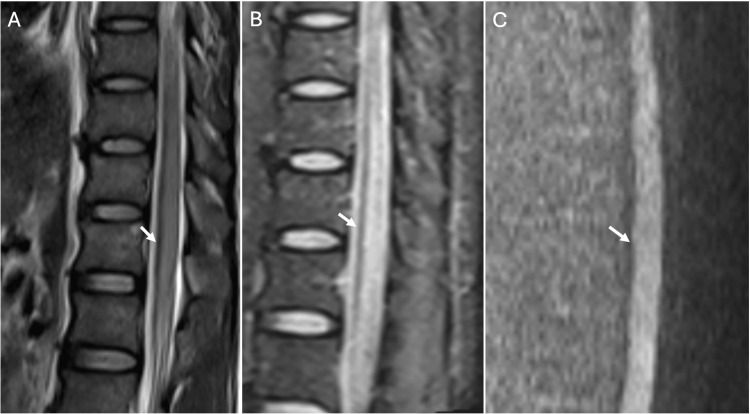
Magnetic resonance imaging (MRI) of the thoracic-lumbar spine, zooming in on the lesion at T9 level. (A) T2-weighted image (T2W) showing hyperintense signal within the spinal cord at the T9-L1 level. (B) Short tau inversion recovery (STIR) image revealing a hyperintense signal, indicating edema. (C) Diffusion-weighted image of the corresponding spinal level showing no restriction of the affected spinal cord, compatible with edema.
